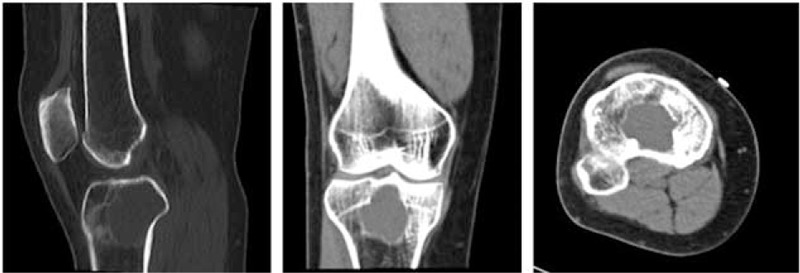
Computed tomography image through the tibial lesion demonstrates lytic lesion within the right proximal tibia has not broken through the cortex, in areas of endosteal erosion, the adjacent cortical bone is sclerotic.
